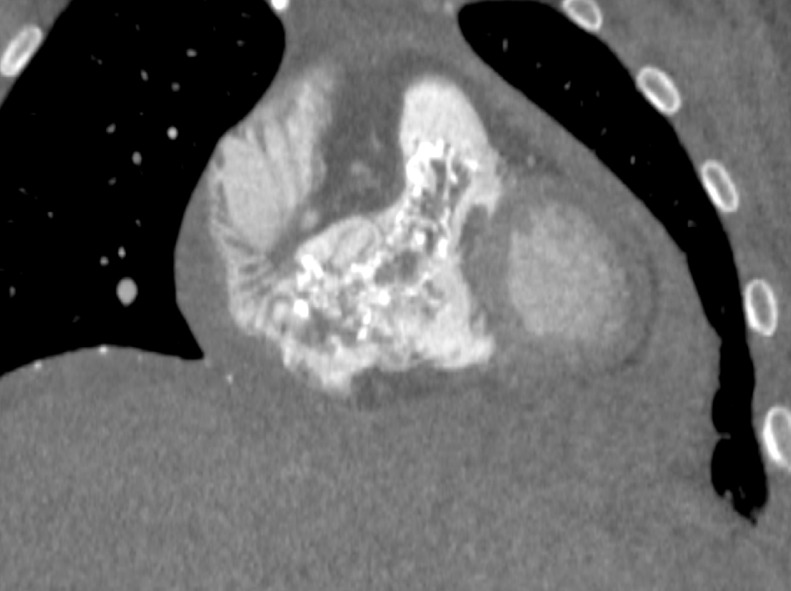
CT heart morphology, coronal reconstruction revealed a heterogeneous irregular calcified mass extending into the right ventricle and pulmonary outflow tract.
